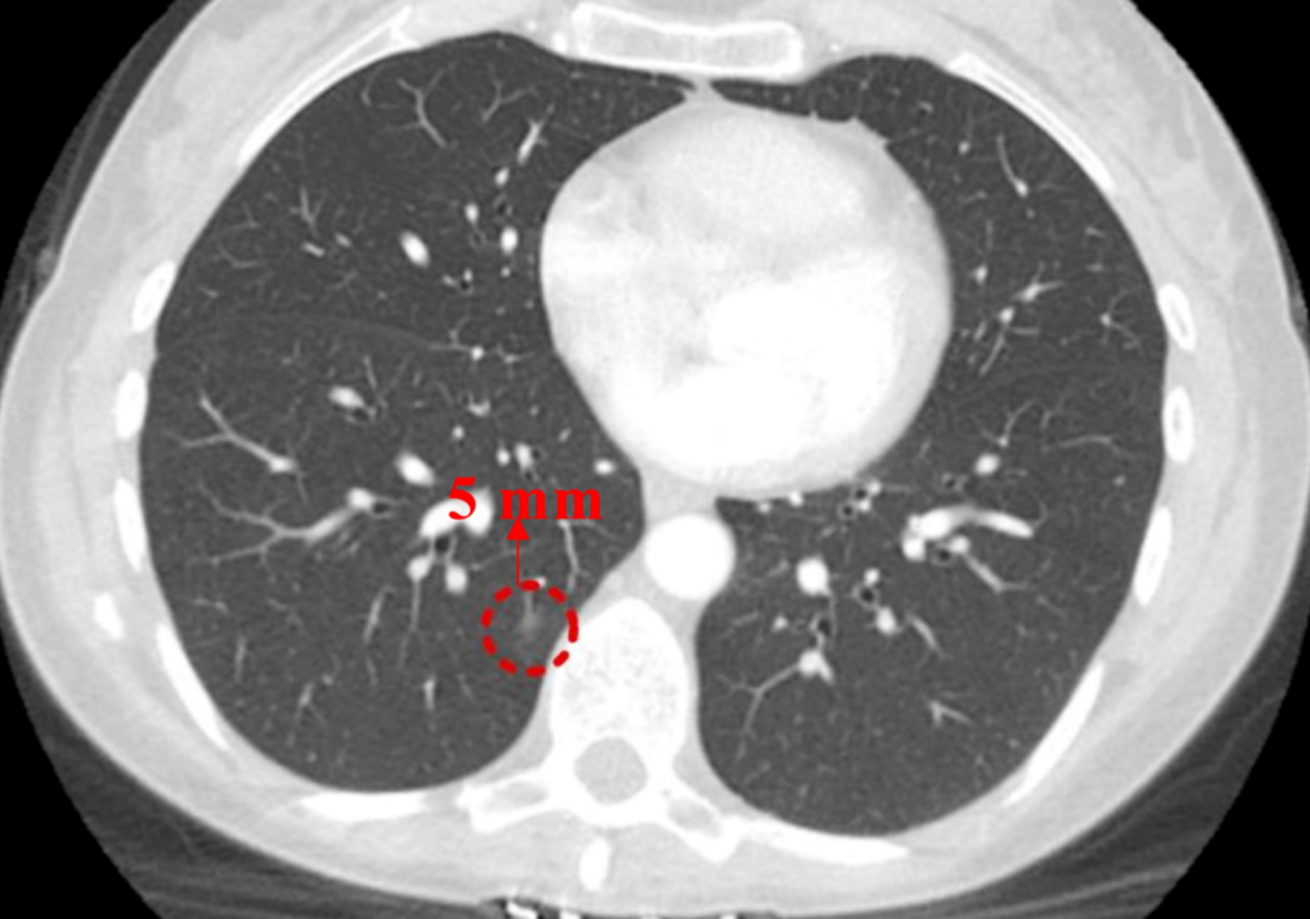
Contrast-enhanced computed tomography (CECT) of the chest (six months from previous CT) New ground-glass opacity (GGO) in the right lower lobe (RLL) measuring 5 mm (appearance concerns for adenocarcinoma with lepidic growth)
